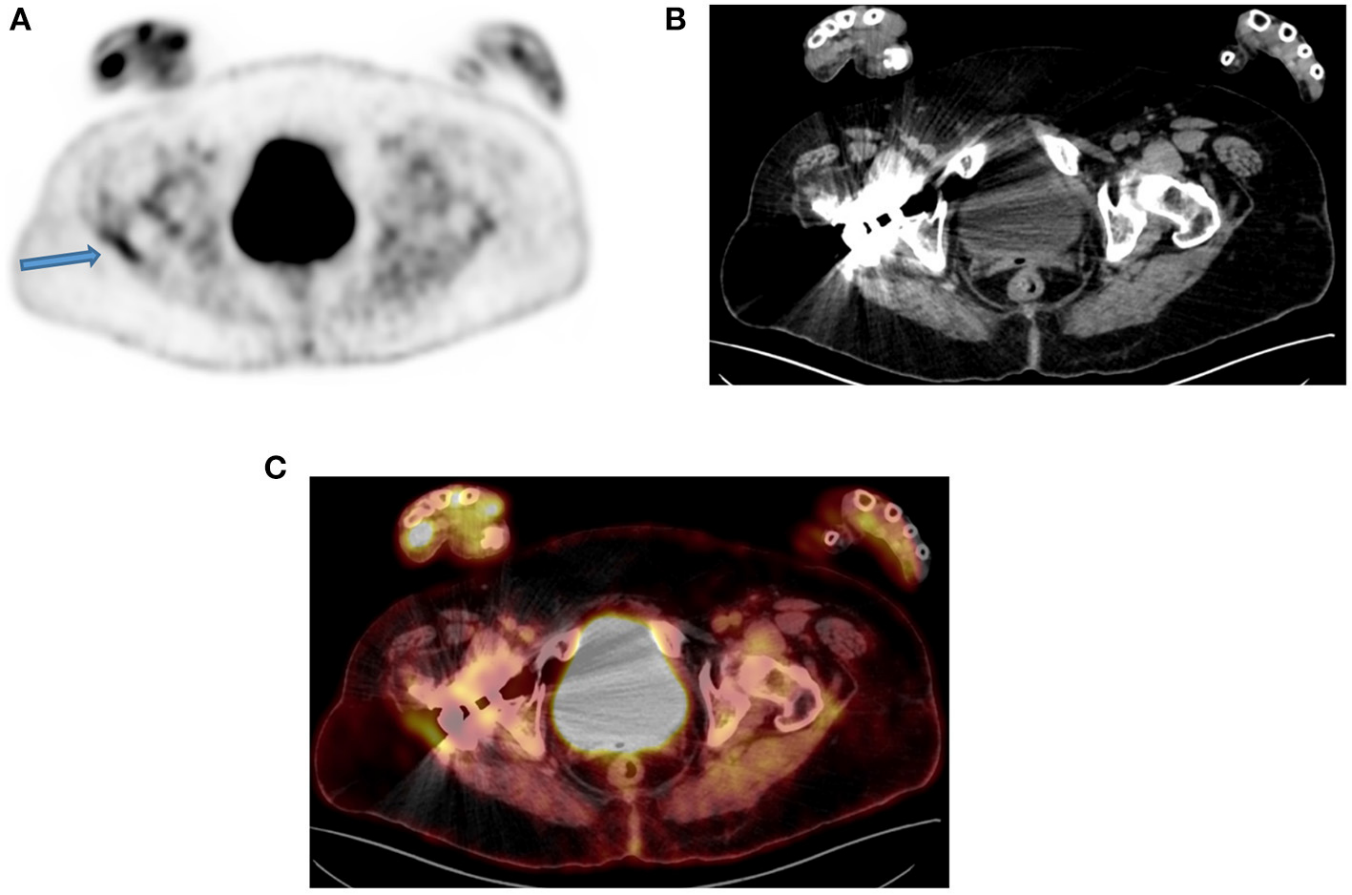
A 50-year-old man with a prosthesis in his right hip join. A PET attenuation-corrected image (A) shows FDG uptake in the lateral aspect of the prosthesis (solid arrow) l) caused by an artifact induced by attenuation correction. Clearly, no corresponding tissue is seen on the CT of the same region, despite the streaks effects from the metal implant (B) and confirmed in the fused PET/CT axial image (C). When not sure on the reason of increased FDG uptake, interpreting physicians must assess the non-attenuation-corrected image of the same region to prevent misinterpretation.
